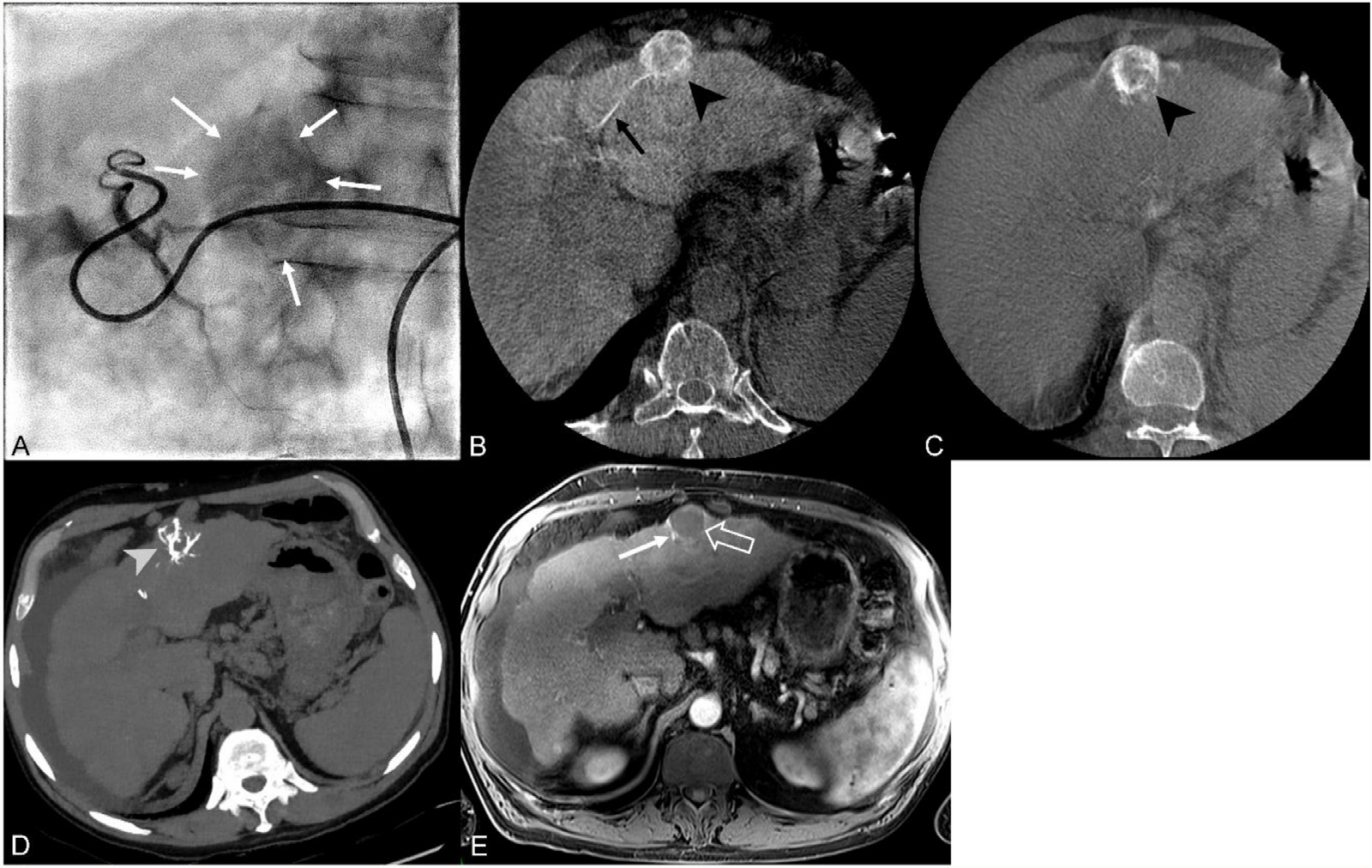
Test group case: a 56-year-old male with two hepatocellular carcinoma (HCC) nodules of the hepatic segments II and III. Manual hyperselective opacification of segment III (A) using a SeQure microcatheter shows a nodular enhancement (white full arrows). The arterial phase of the contrast enhanced cone beam CT (CBCT) B) shows the feeding artery (black full arrow) and the enhanced subcapsular nodule (black arrowhead). The result of the following drug eluting beads transarterial chemoembolization (DEB-TACE) is a G1 fixation of the nodule at H0 CBCT (C – black arrowhead) with persistent fixation at the M1 non enhanced CT (D – black arrowhead) displayed in maximal intensity projection (MIP). Devascularization of the nodule is shown at the M1 MRI (E – white hollow arrow) with a thin enhancing rim (white full arrow) that disappeared in following controls.
